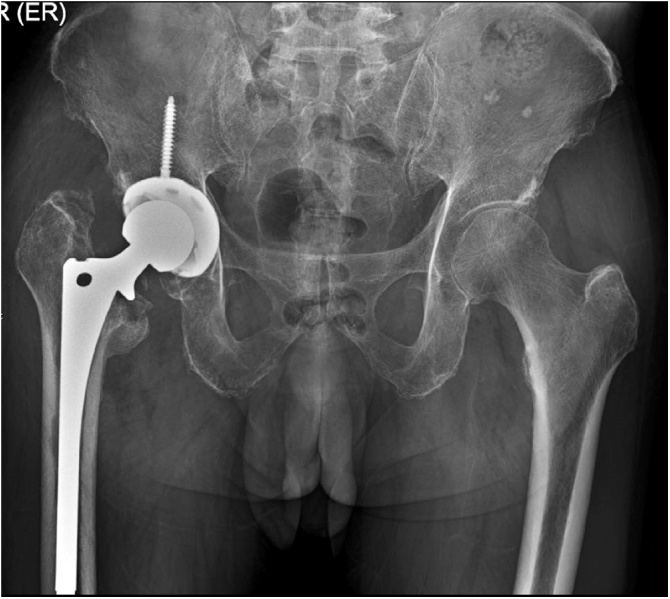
Plain radiographs at 6 months after revision total hip replacement.
